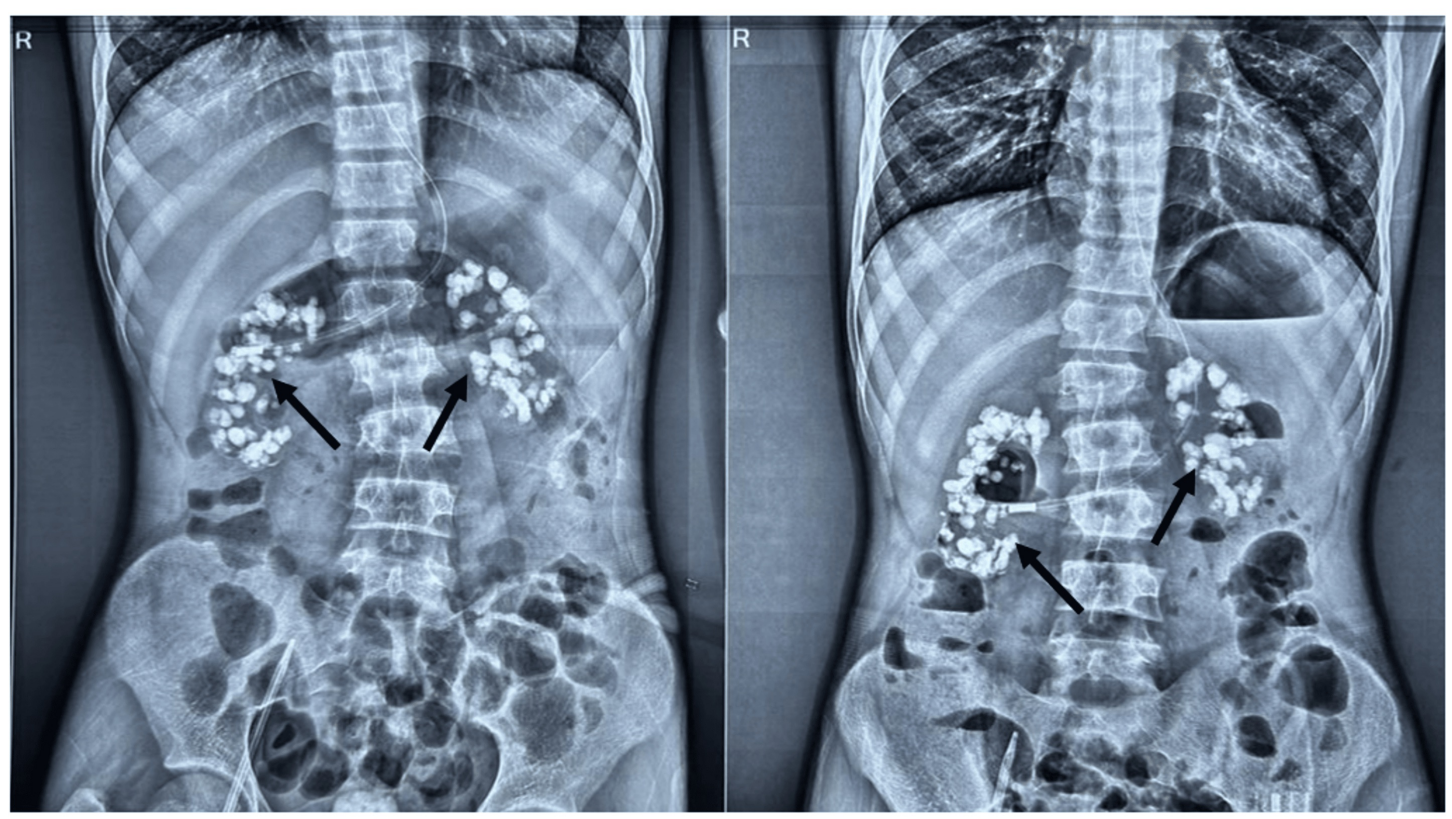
X-ray abdomen film of our patient showing bilateral kidney stones (marked by black arrows).
